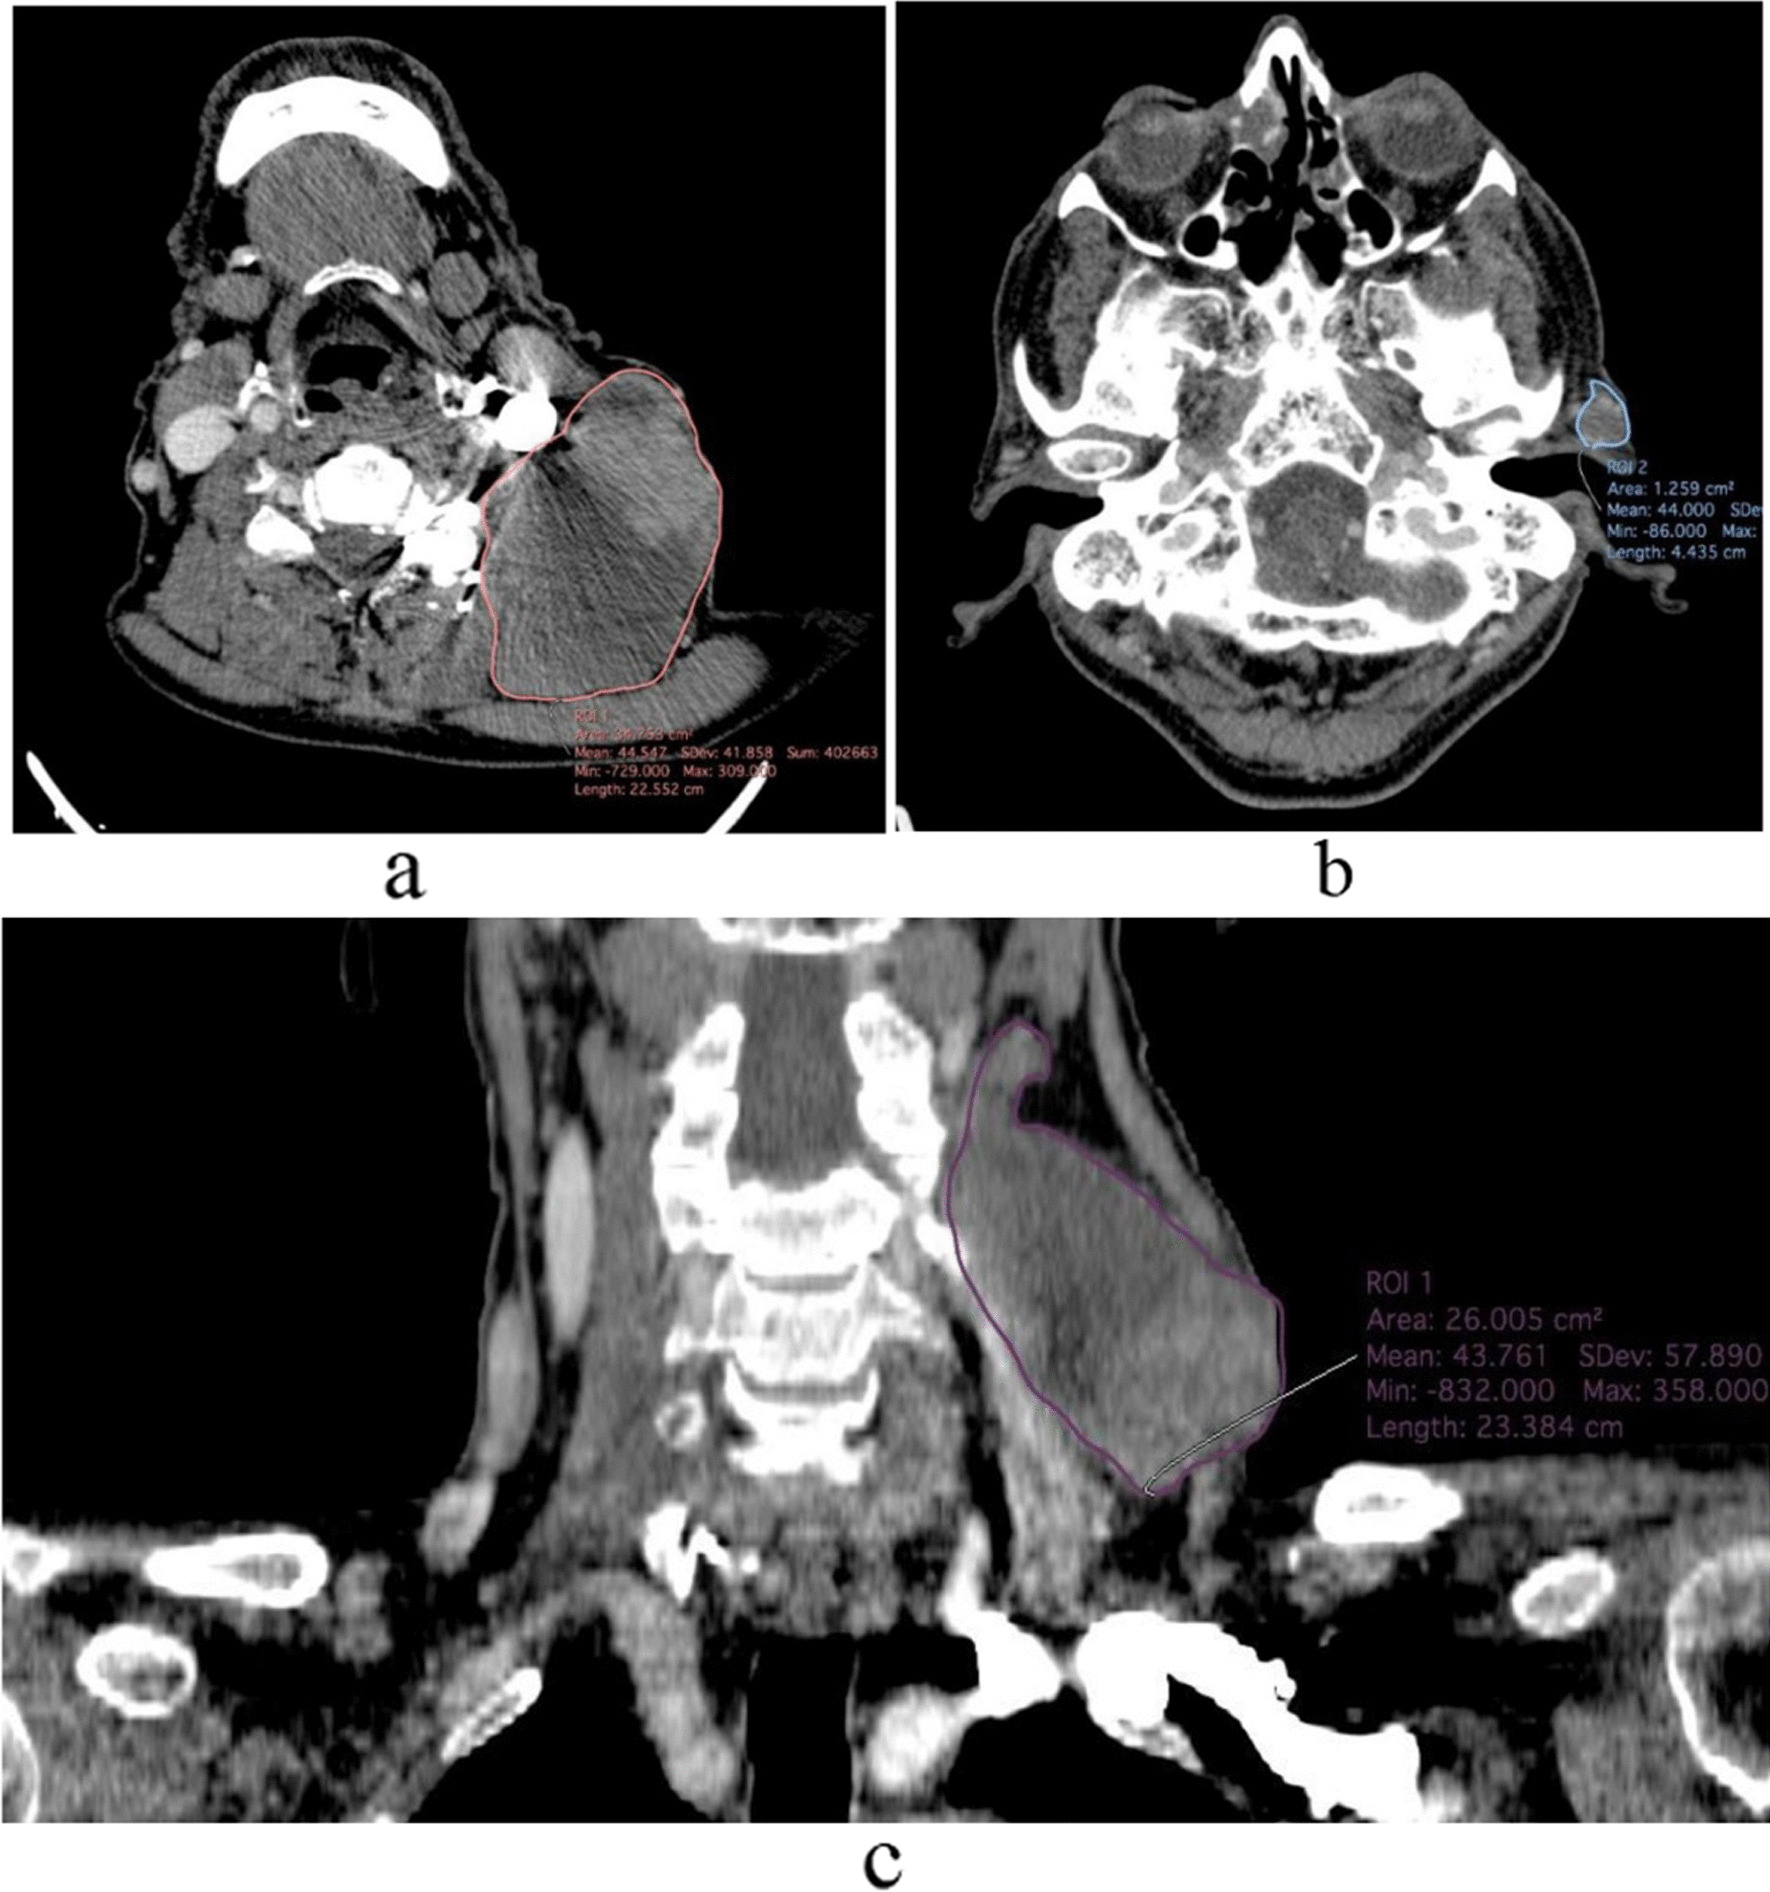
Post contrast neck computed tomography scan; axial and coronal reformat images, demonstrates a very large cystic cervical mass (46 × 23 mm) with papillary projections in its antero-lateral aspect, in left neck zone Vb (a, c). Moreover, there is a small heterogeneously enhancing nodule with small central cystic component in left pre-auricular region, in the site of prior parotidectomy (b)
